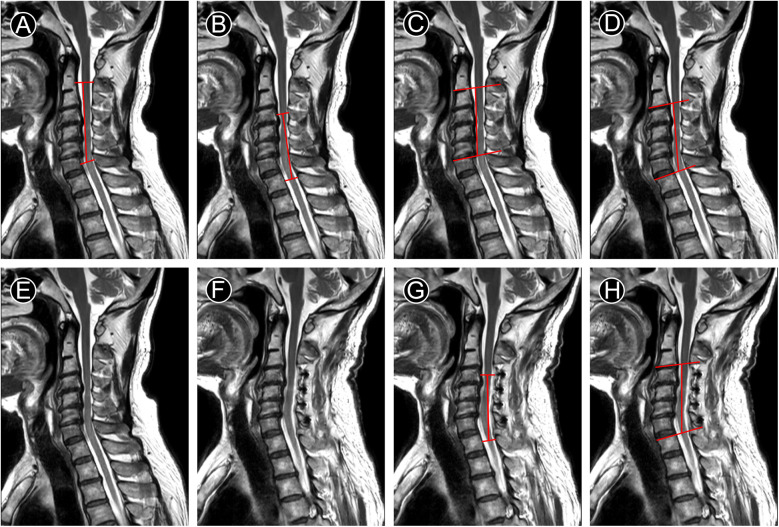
T2-MRI of a case with MCSM underwent LAMP for C4–C7. (A–D) preoperative measurement line. (G–H) postoperative measurement line. A. K-line(−), (B) mK-line(+), (C) SC-line Type III, (D) mSC-line(+), (E) preoperative image shows obstructed cerebrospinal fluid, (F) Image at 7 months after operation shows unobstructed cerebrospinal fluid, (G,H) mK-INT and mSC-INT increased.
